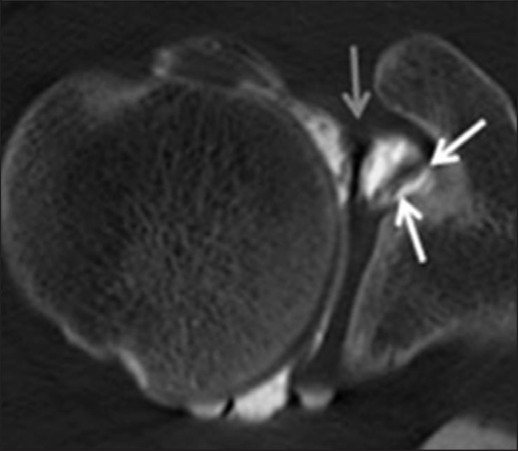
Coracoglenoid ligament is demonstrated on a superior axial CTA image (white arrows). It arises from the supraglenoid tubercle, covering the top of the glenoid rim and superior labrum to insert on the middle of the coracoid process. The superior glenohumeral ligament is indicated by the grey arrow.
